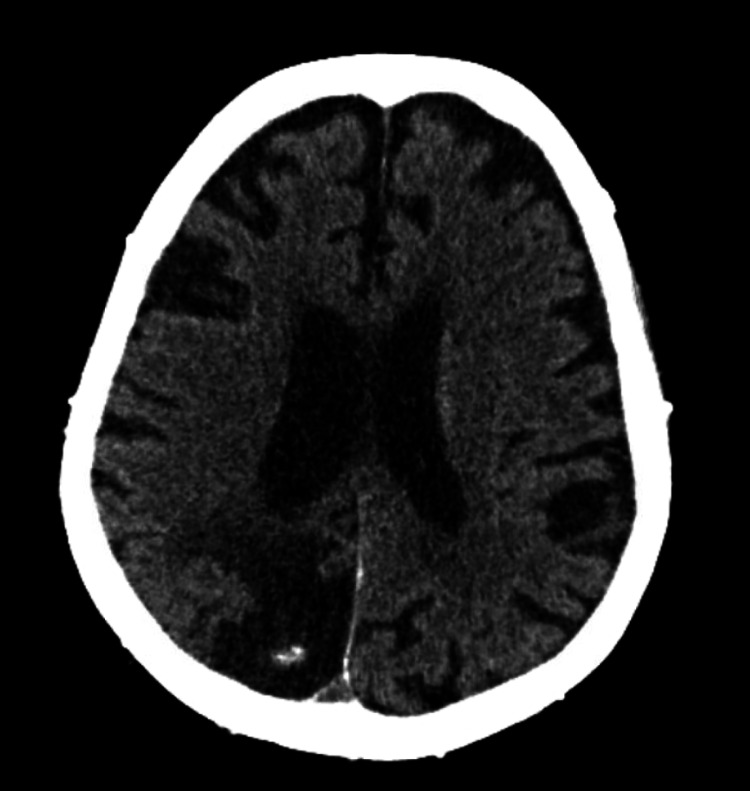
CT head without contrast
